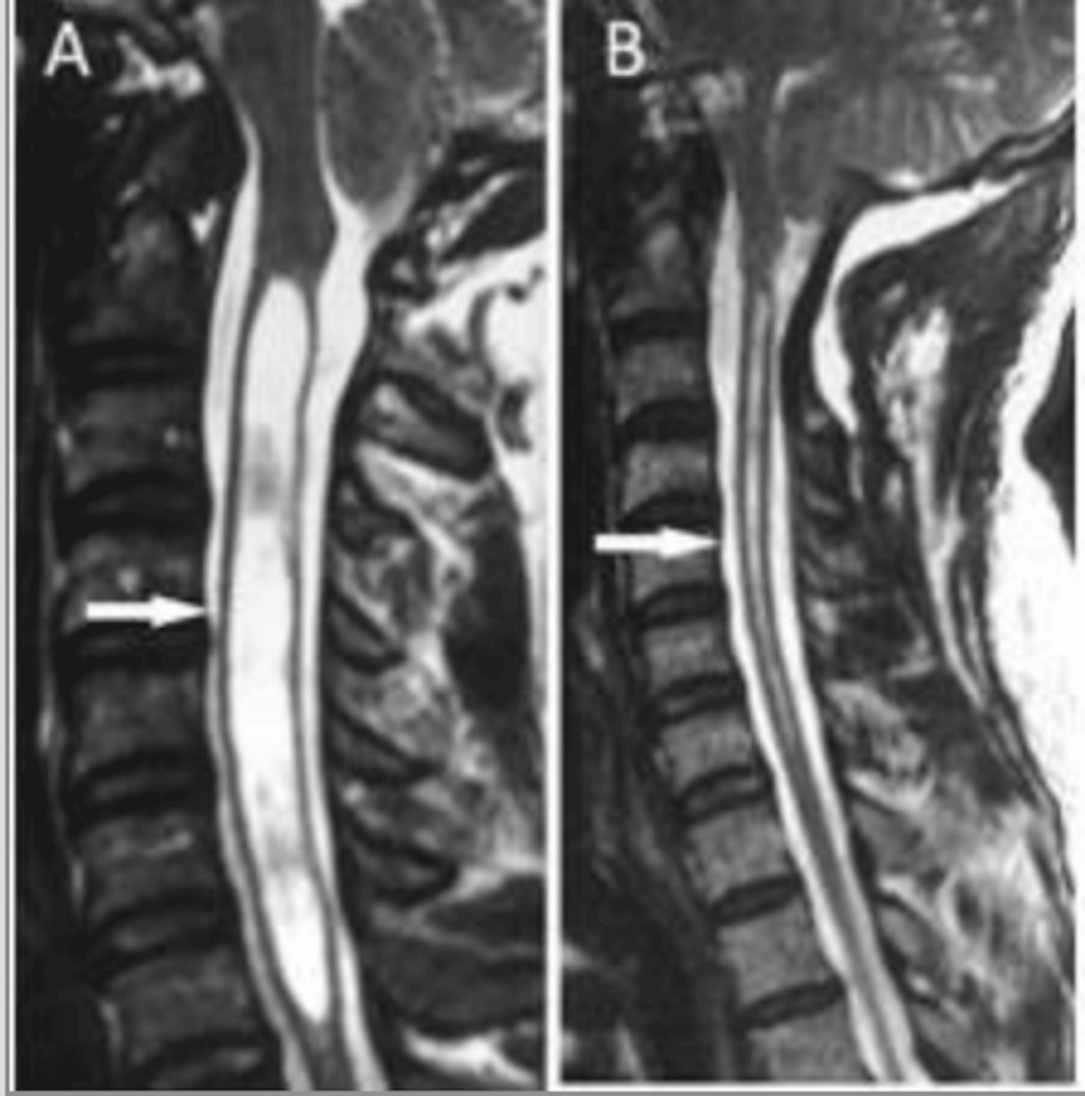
Pre- and postoperative sagittal T2-weighted MRI in a patient with CM-I and syringomyelia. (A) Preoperative image showing a prominent syrinx (arrow). (B) Postoperative image obtained 12 months after surgery showing reduction in syrinx size and restoration of CSF flow Image reproduced from Open-i, National Library of Medicine (NIH) [30] (CC BY 2.0 Deed). MRI: magnetic resonance imaging; CM-I: Chiari malformation type I; CSF: cerebrospinal fluid
